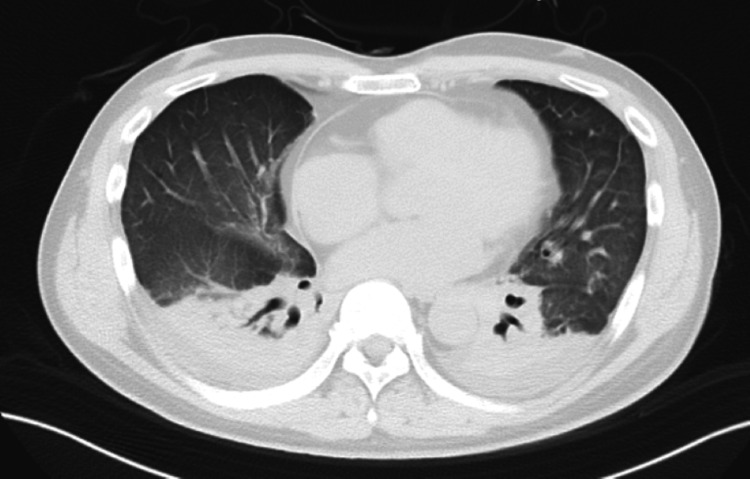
Chest CT on admission Axial chest CT image of a patient with ARDS secondary to *Salmonella septicemia*. The image reveals bilateral ground-glass opacities and patchy consolidations, predominantly in the posterior and basal lung regions. Bilateral mild pleural effusions are also noted. CT: computed tomography, ARDS: acute respiratory distress syndrome
